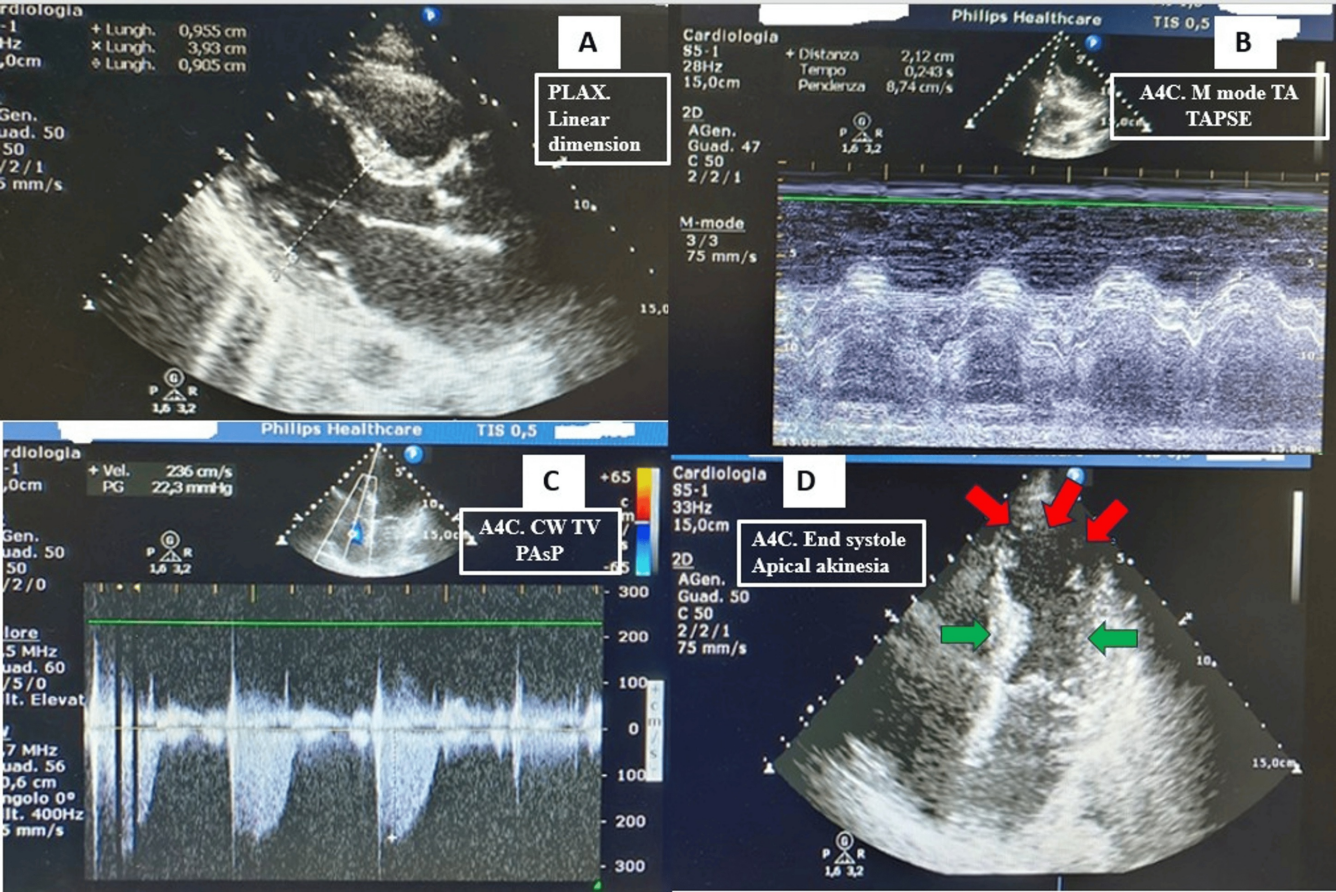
Echocardiography performed the day after angioplasty. (A) PLAX view showing normal wall thickness and normal intracavitary dimensions. (B) A4C M-mode at the TA demonstrating TAPSE within normal limits (21 mm). (C) A4C CW Doppler across the TV showing normal estimated PASP of 25 mmHg (22 mmHg + RAP 3 mmHg). (D) A4C end-systolic frame showing apical akinesia (red arrows) with preserved contraction of the basal segments (green arrows). PLAX: parasternal long-axis view; A4C: apical four-chamber view; TA: tricuspid annulus; TAPSE: tricuspid annular plane systolic excursion; CW: continuous wave; TV: tricuspid valve; PASP: pulmonary artery systolic pressure; RAP: right atrial pressure
